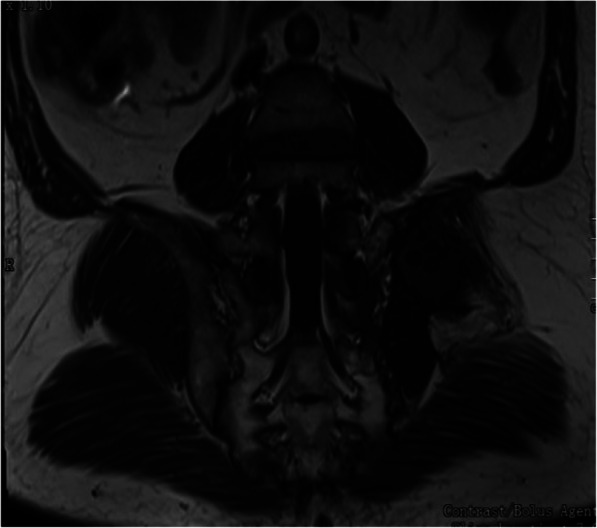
MRI coronal plane T1, which showing irregular low signal intensity of left ilium and iliopsoas muscle,and multiple circular lower signal intensity shadows can be seen in the low signal intensity shadow of the left iliac bone and iliopsoas muscle
